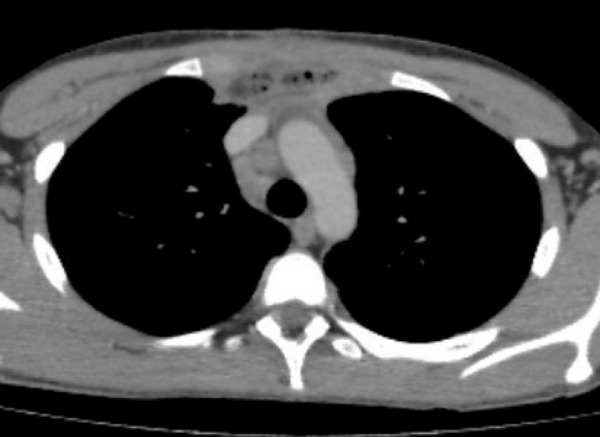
Figure 2:Postoperative CT scan.
